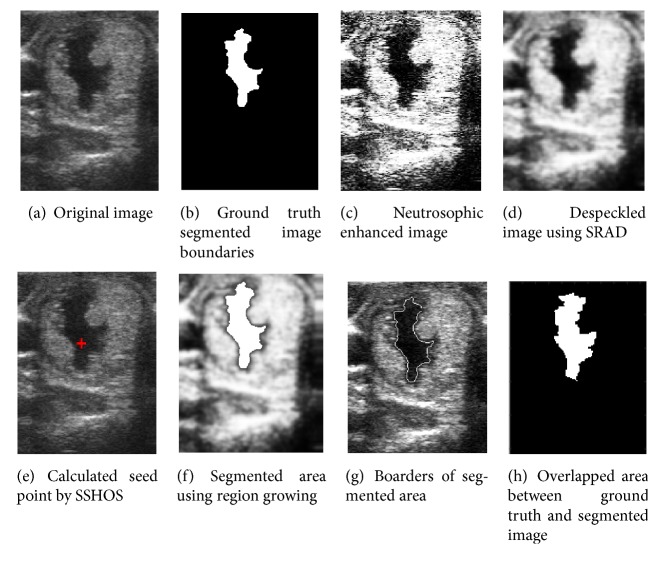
Ultrasound image (image 31M).
